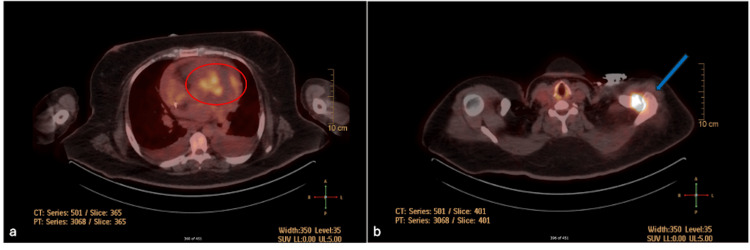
Positron emission tomography scan (a) Fluorodeoxyglucose (FDG)-avid activity in the anterior septum, mid-lateral ventricular wall, and pulmonic valve suggestive of focal endocarditis (red circle). (b) Intense FDG uptake at the level of the left acromioclavicular joint suspicious for possible septic arthritis (blue arrow).
